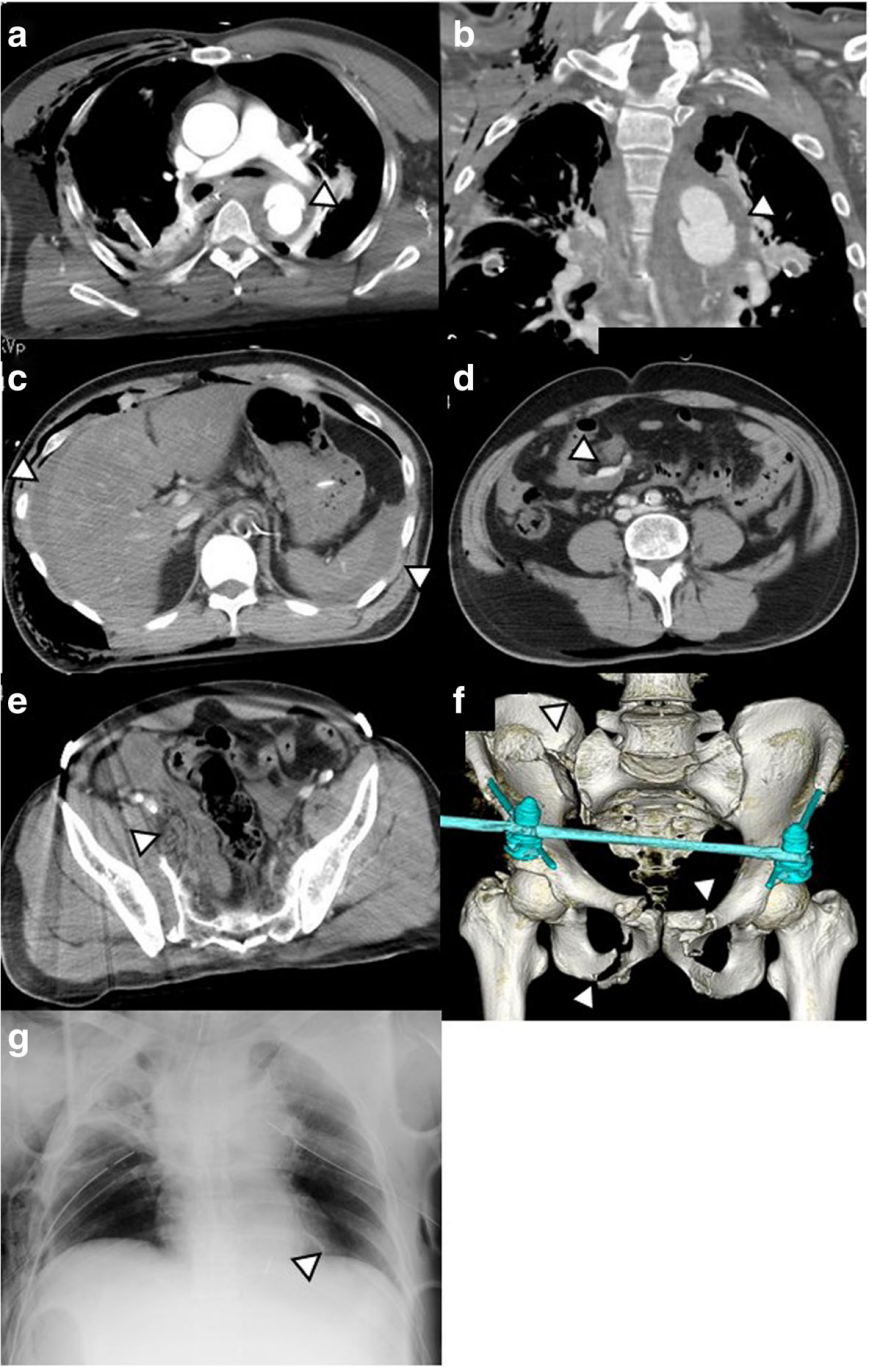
Chest radiography and computed tomography on initial resuscitation. a, b Thoracic injury to the aorta. c Hemoperitoneum. d Extravasation from the mesenteric injury. e Retroperitoneum from the pelvic fracture. f Fracture of the pelvis shown from reconstructed computed tomography images, and g position of resuscitative endovascular balloon-occlusion of the aorta (REBOA), with the *marker* indicating the top of the REBOA
